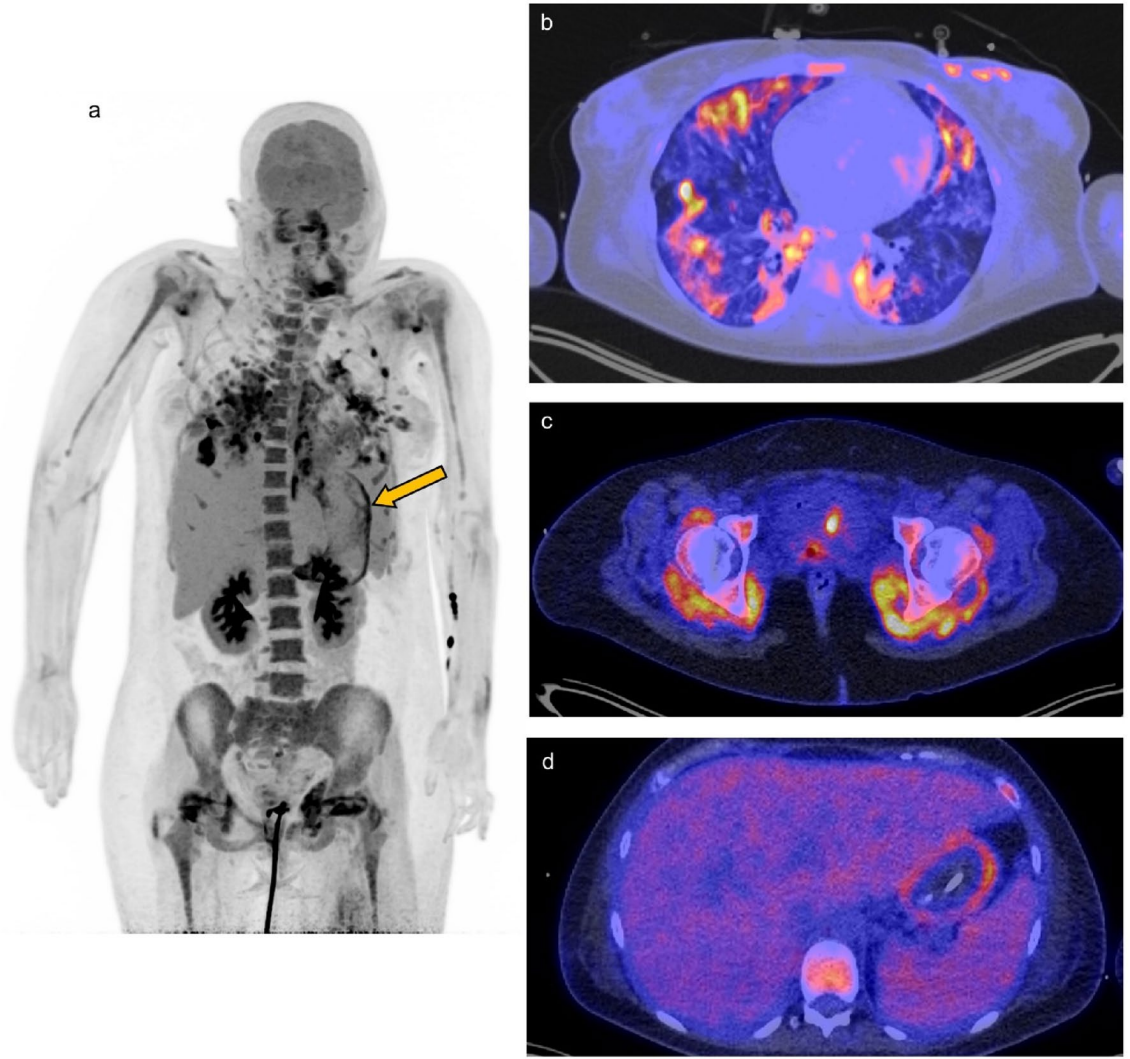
[^18^F]FDG-PET/CT of patient 4. The last patient is a 26-year-old pregnant female who was admitted because of mild respiratory failure due to COVID-19 and premature contractions wherefore a C-section was performed. Two days after C-section, the patient became severe respiratory insufficient and was mechanically ventilated. Due to a second episode of respiratory decline and ongoing inflammation, an [^18^F]FDG-PET/CT was performed. A gastritis was found as a possible secondary infection focus. The patient was still SARS-CoV-2 positive at the time of [^18^F]FDG-PET/CT. a Maximum intensity projection. Physiological uptake in the kidneys and liver. Reduced uptake in the brain due to sedation. Reactive bone marrow. The yellow arrow points toward the uptake in the gastric wall. b Transaxial fusion PET/CT image of the lungs with ground-glass opacities and some consolidations on CT, characteristic for COVID-19 ARDS. Heterogenous uptake of [^18^F]FDG. CT in lung setting. c Transaxial fusion PET/CT image of the hips with high uptake in the posterior lateral and medial muscle of the hip at both sides. CT in soft tissue setting. d Transaxial fusion PET/CT image of the abdomen with clear [^18^F]FDG uptake in the gastric wall. *SUV-bw threshold of 5.00 α* = *50%*
